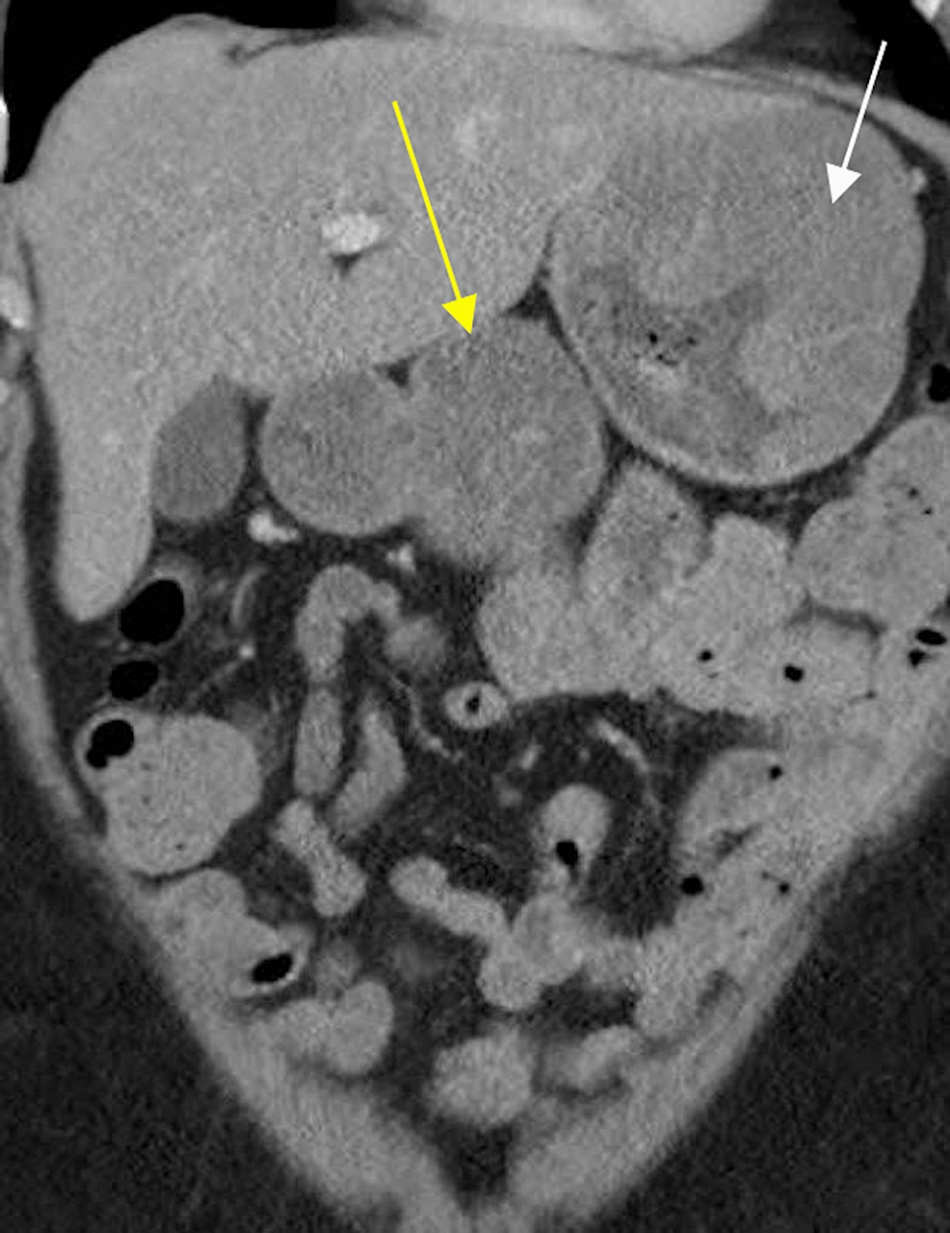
Coronal IV contrast-enhanced CT image demonstrates marked gastric wall thickening secondary to extensive rugal fold hypertrophy (white arrow). The antrum does not demonstrate wall thickening (yellow arrow).
